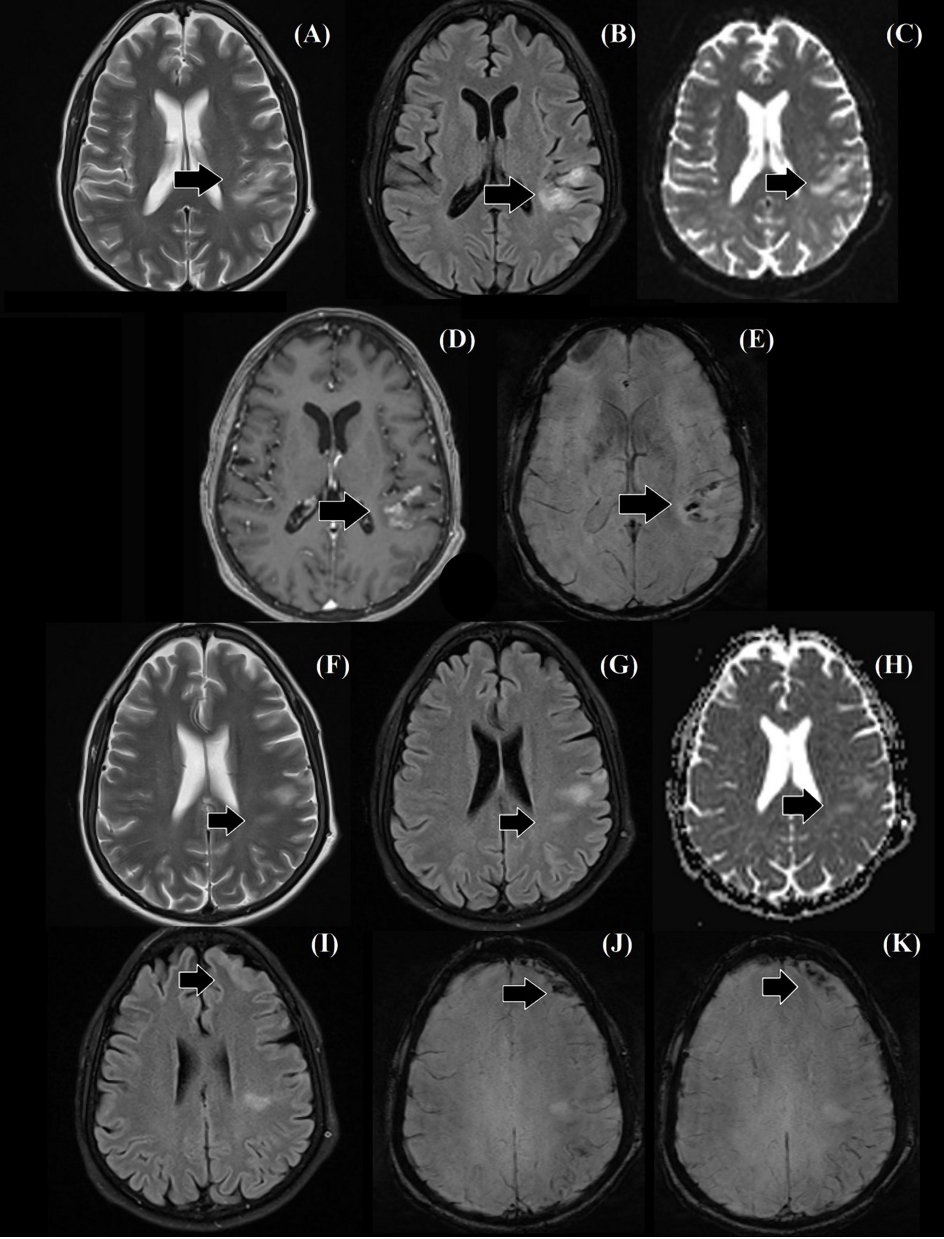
MRI scan of the brain, axial cross-section images. A–C) T2WI, FLAIR and DWI sequences showing the ischemic lesion in the left temporoparietal region. D, E) T1WI and SWI sequences revealing the hemorrhagic conversion of the lesion. F–H) T2WI, FLIAR and DWI sequences revealing a second ischemic lesion in the posterior area of the left parietal lobe. I–K) FLAIR and SWI sequences showing a convexal subarachnoid hemorrhage in the left frontal lobe. T2WI – T2 weighted image; FLAIR – Fluid attenuated inversion recovery; DWI – diffusion-weighted imaging.
